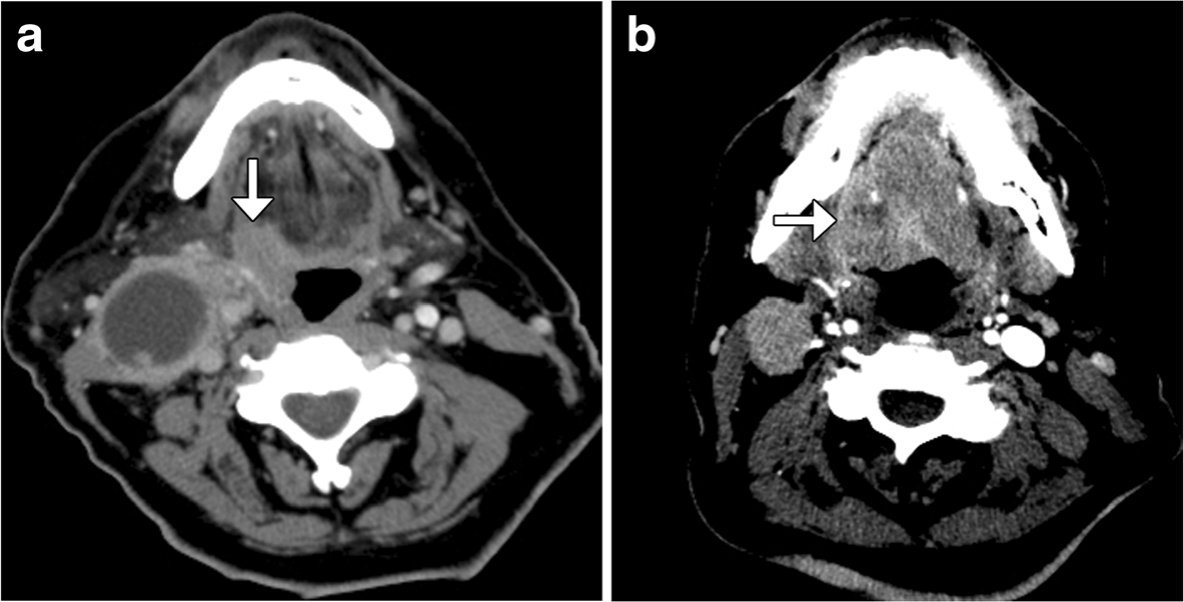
HPV-positive versus HPV-negative oropharyngeal squamous cell carcinoma. Axial CT image (a) shows a well-defined right oropharyngeal mass (arrow) and large cystic right cervical lymphadenopathy in a patient with HPV-positive squamous cell carcinoma. Axial CT image (b) shows an infiltrative right oropharyngeal mass (arrow) and solid right cervical lymphadenopathy in a patient with HPV-negative squamous cell carcinoma
